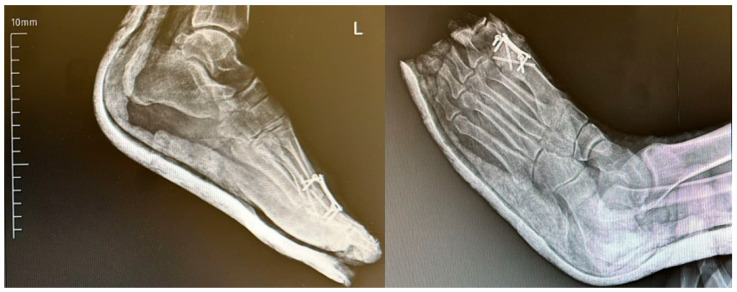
Lateral radiographic view demonstrating optimal dorsiflexion angle following first metatarsophalangeal (MTP) joint arthrodesis. L: Left.
